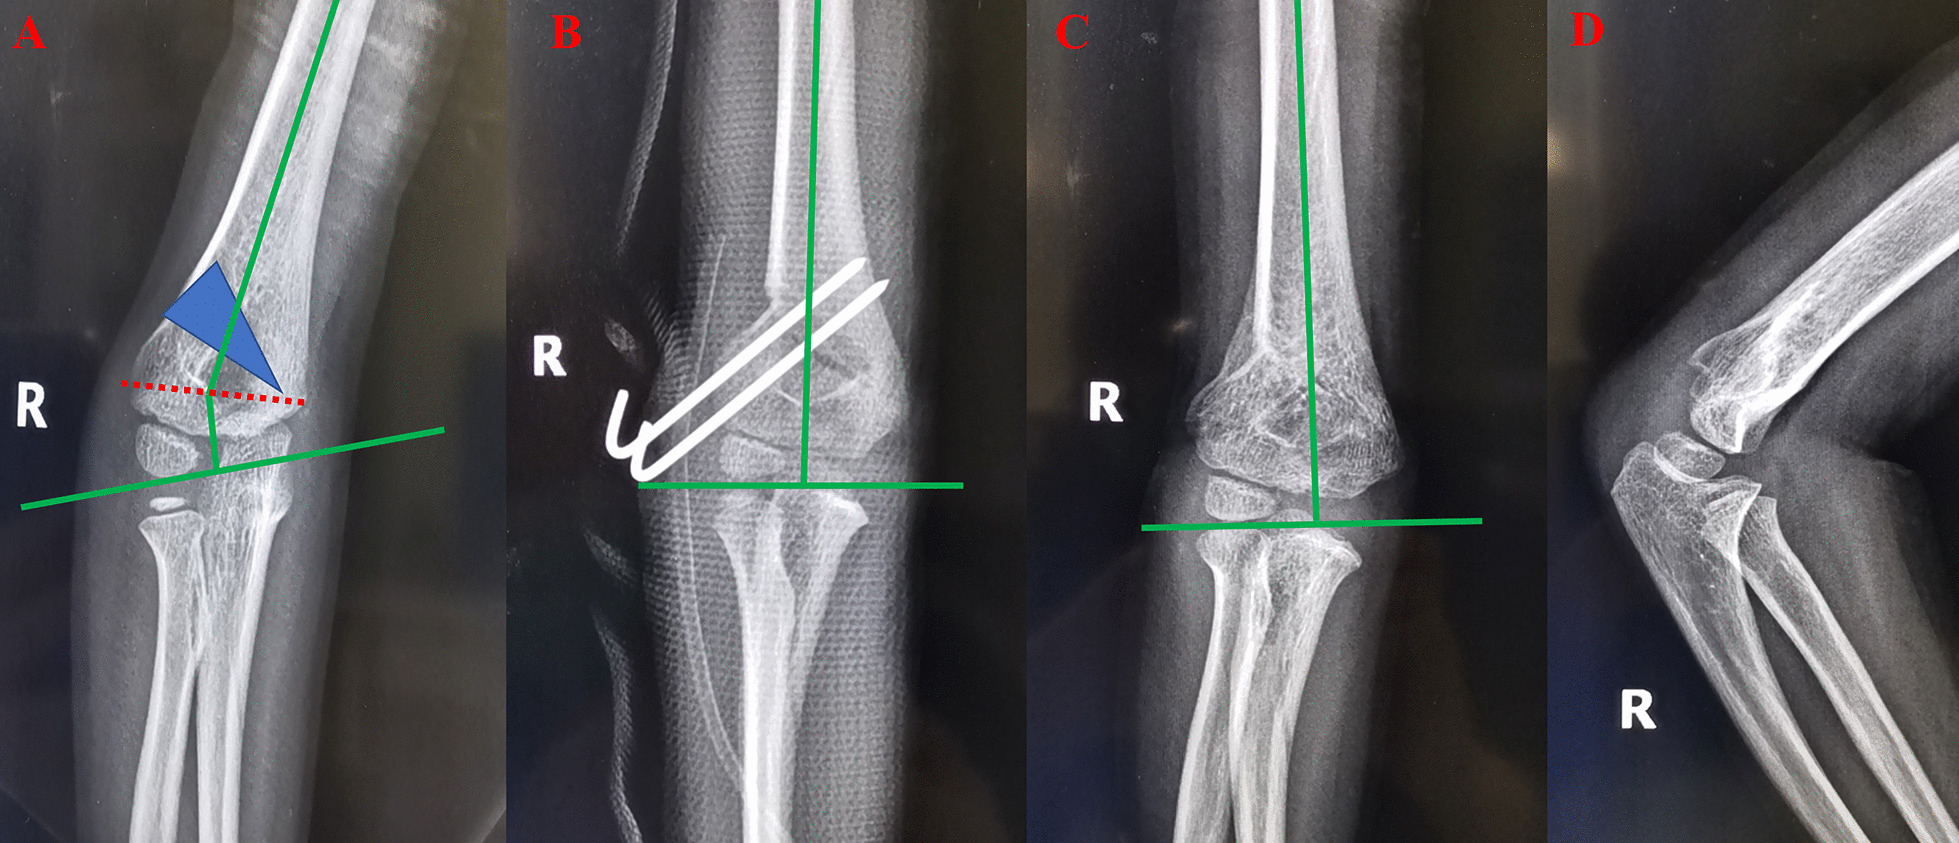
Representative case of a 6-year-old male with right cubitus varus deformity. A Shows the design of the osteotomy. B Shows antero-posterior radiographs of the osteotomy on the 2nd postoperative day. C, D Show the antero-posterior and lateral sides of the patient at 3 months postoperatively
